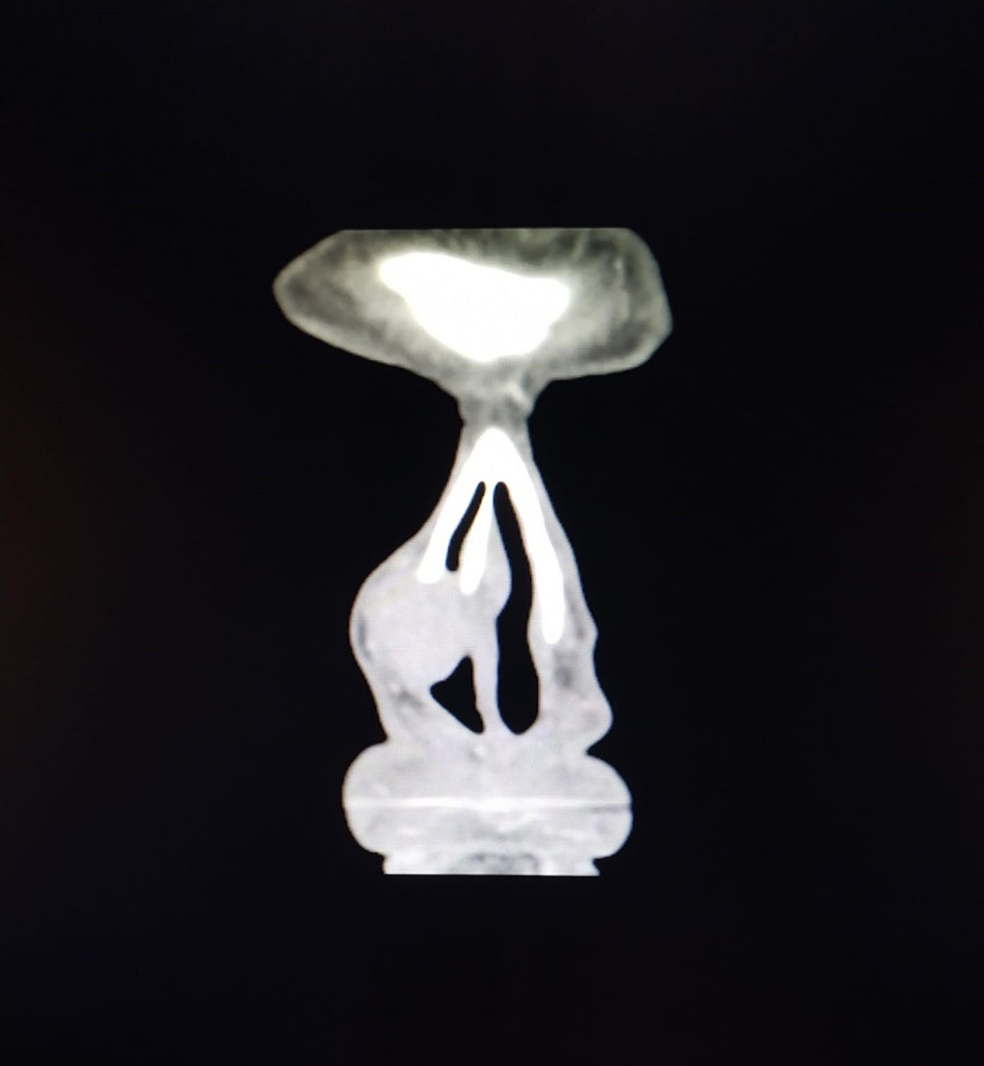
CT scan - coronal view CT scan showing a homogeneous nodular mass of soft tissue with the erosion of the frontal maxillary apophysis extending into the nasal vestibule CT: computed tomography
